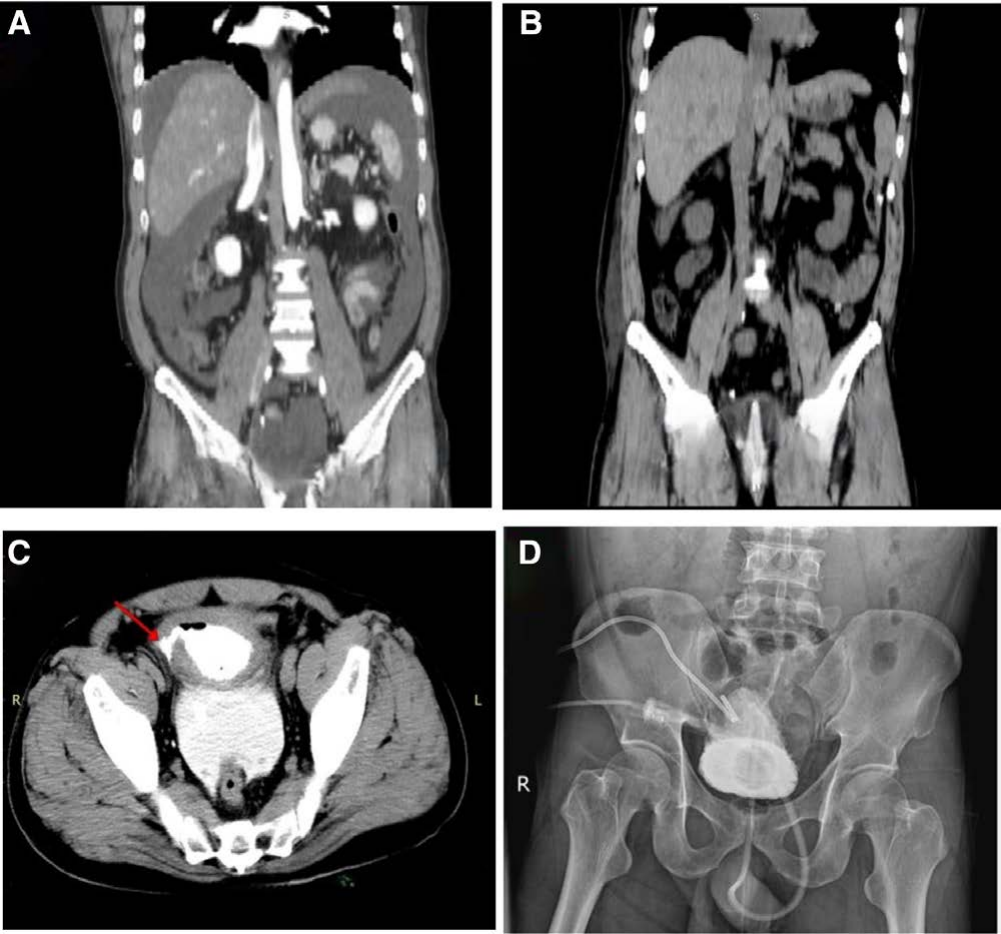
CT imaging of spontaneous bladder rupture. (A) Admission non-contrast abdominal CT demonstrating large-volume ascites. (B) Follow-up abdominal CT 3 days after bladder repair and catheterization showing near-complete resolution of ascites. (C) Abdominal CT after retrograde cystography with intraperitoneal contrast extravasation (arrow) and intravesical gas. (D) Fluoroscopic image during retrograde cystography confirming contrast leak.
